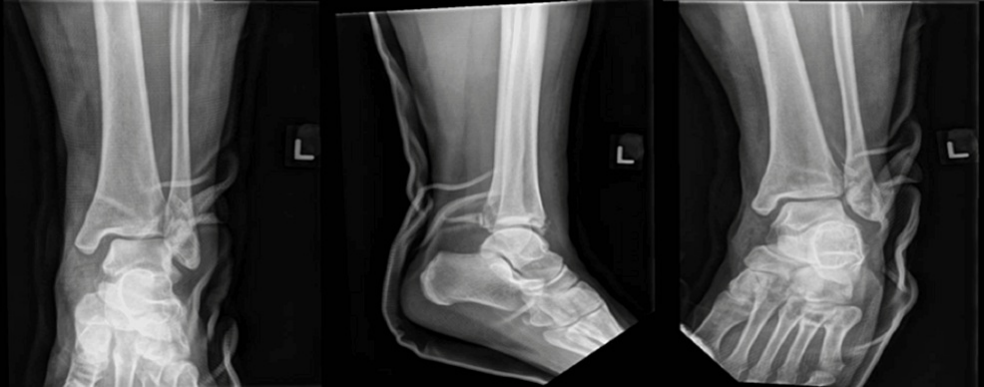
Post manipulation and reduction left ankle mortise/lateral/anterioposterior X-ray images
